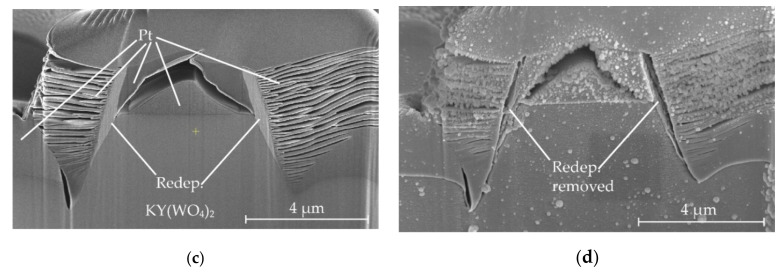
(a) A strip of sidewall redeposition that has delaminated during ultrasound cleaning and curled up due to internal shear stresses. (b) Sidewall redeposition that has been partially removed during an insufficiently long HCl etching step. (c) Cross-section of a structure with significant redeposition, covered with a thick layer of platinum. (d) The same cross-section after a 5 min HCl etching step. Most of the redeposited material is removed.
